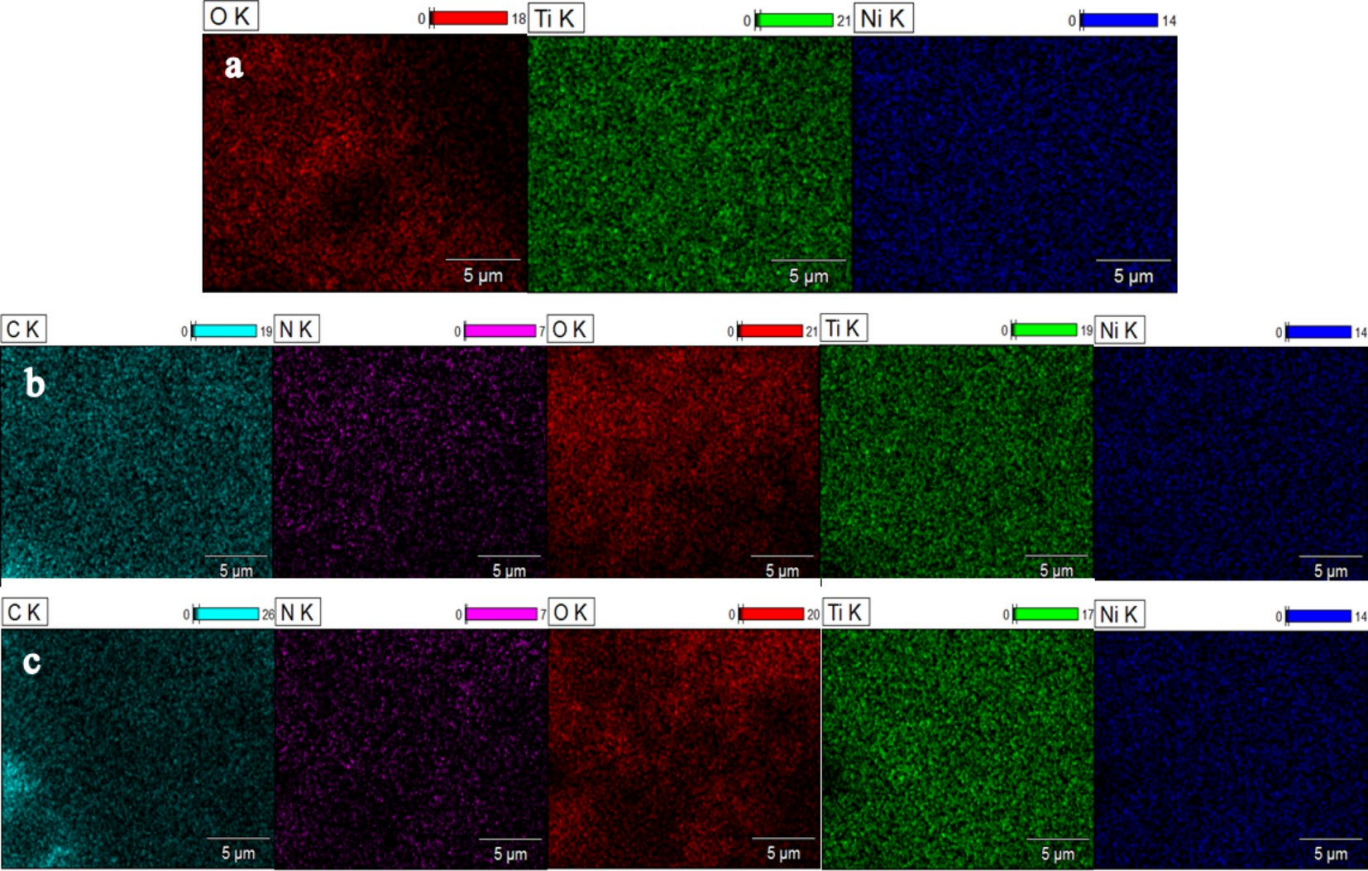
Energy dispersive X-ray mapping of: (a) NiTiO_3_, (b) 1wt.% PANI@NiTiO_3_, and (c) 2wt.% PANI@NiTiO_3_.
